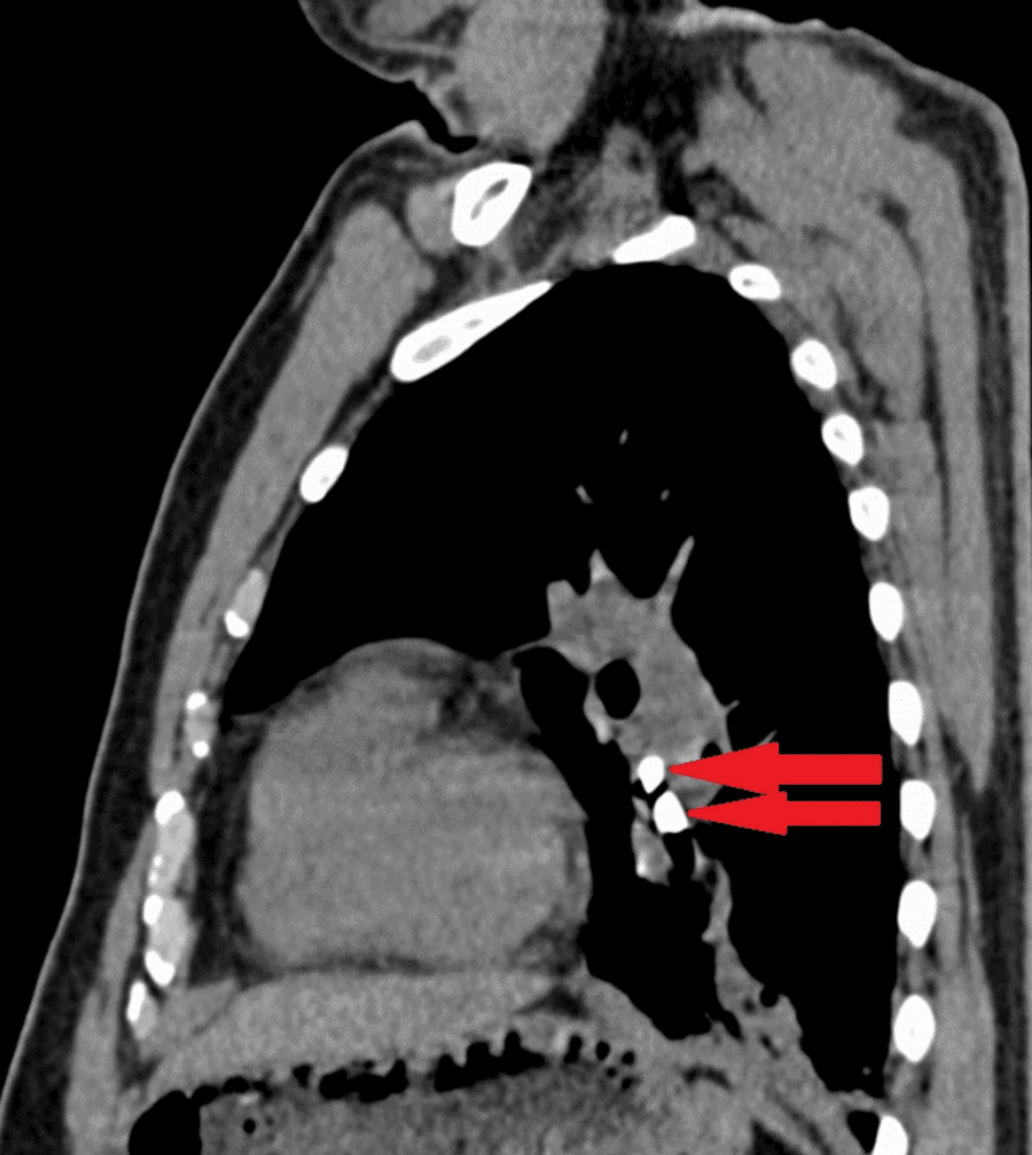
High-resolution computed tomography of the chest mediastinal window sagittal section revealed two well-defined bone density areas (HU+1850 to +1980) seen within the left lower lobe bronchus, one measuring 11 x 8 mm and the other measuring 8 x 6 mm (red arrows).
